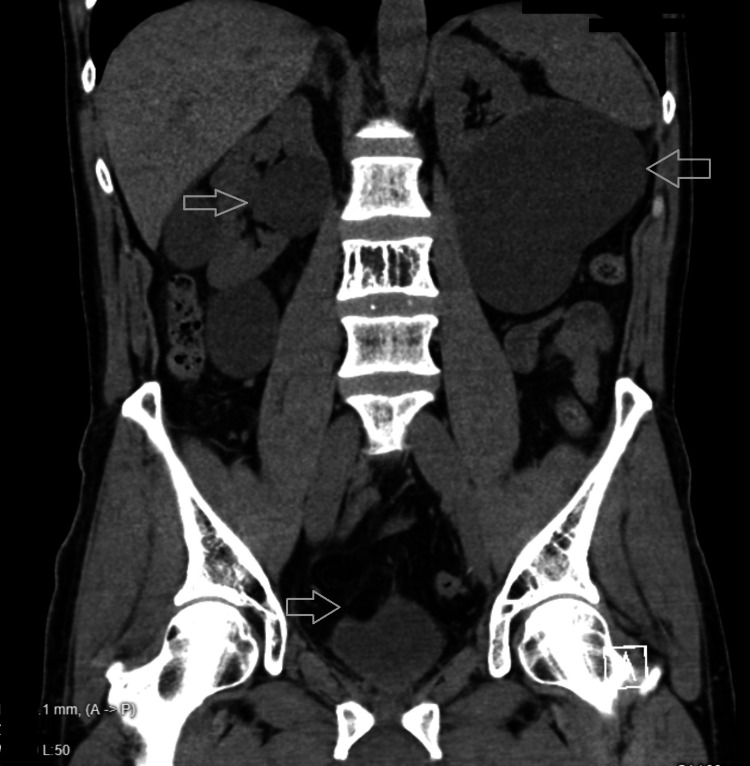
CT abdomen and pelvis without contrast (coronal view) CT: computed tomography
